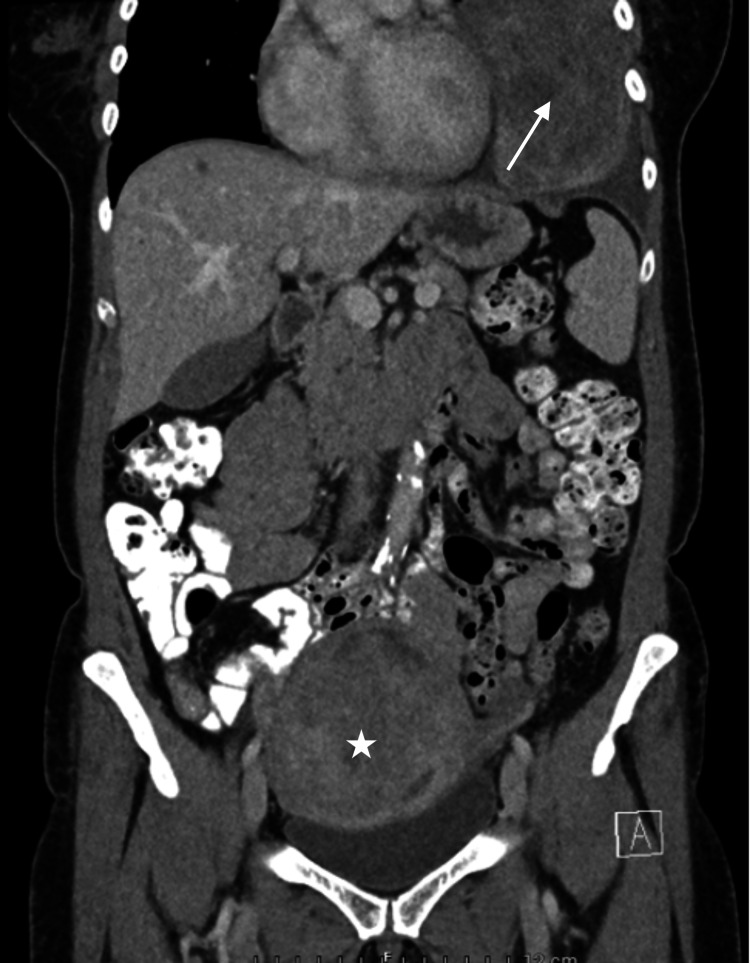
CT of the abdomen showed a uterine mass. No metastases. Coronal plane. White star: uterine mass; white arrow: pleuropulmonary solitary fibrous tumour (SFT)
